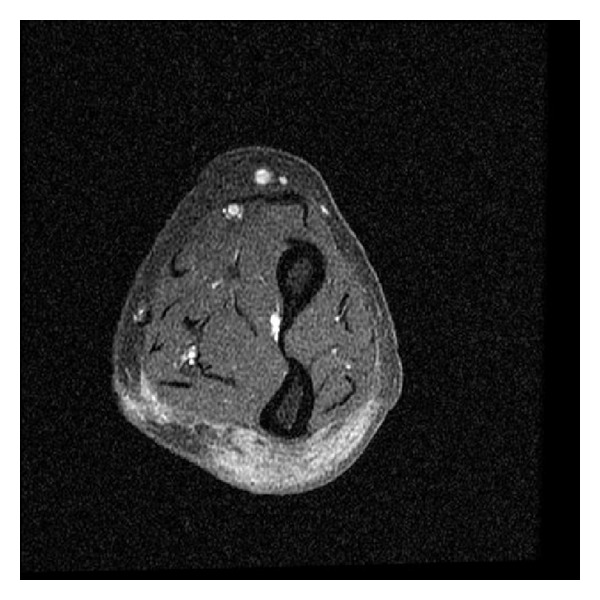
MRI scan (T1-weighted image) of the forearm. The image shows an area of low intensity at the subcutaneous level.
